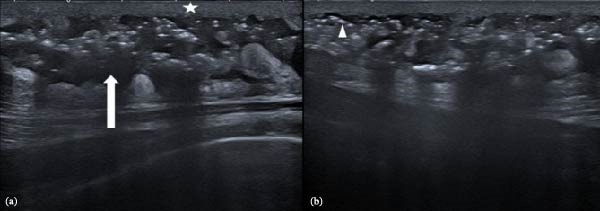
Neck ultrasonography images of a 58‐year‐old patient with deep neck infection. (a) The asterisk indicates diffuse skin thickening, and the white arrow demonstrates reticular subcutaneous edema. (b) The arrowhead highlights one of the multiple hyperechoic foci with comet‐tail artifacts, consistent with subcutaneous gas.
